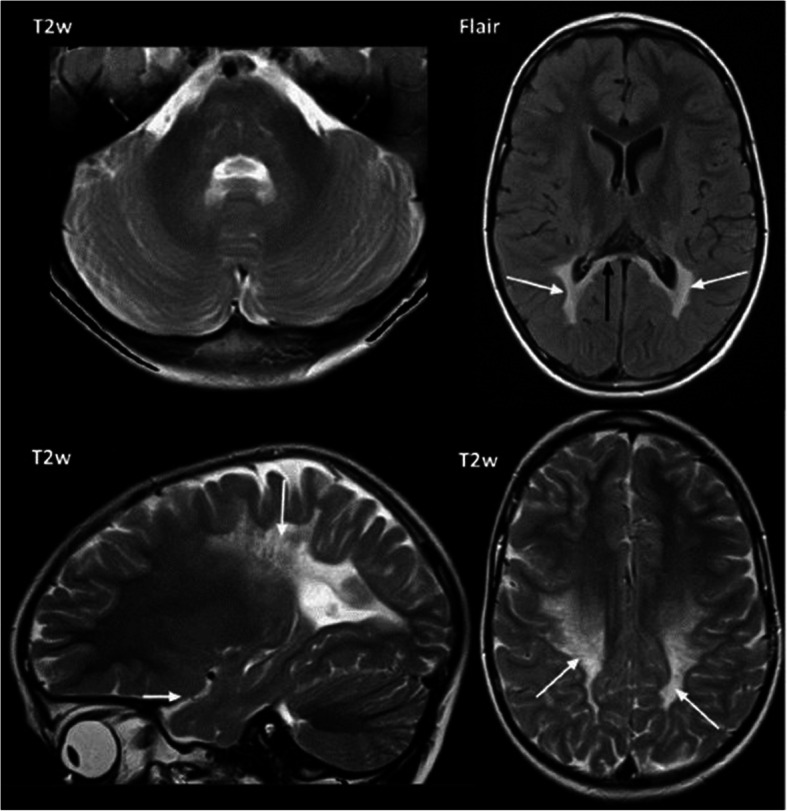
Pattern B1: MR images of a ‚later onset‘patient. Patient 34 (onset 5 years, age at MRI 5 years 1 month). White matter is primarily affected in the periventricular parieto-occipital regions (arrows in the upper right Flair image, and lower left sagittal and lower right T2w images). The Flair image shows the involvement of the splenium of the corpus callosum (black arrow). Cerebellar white matter is not affected (axial T2w image upper left).
